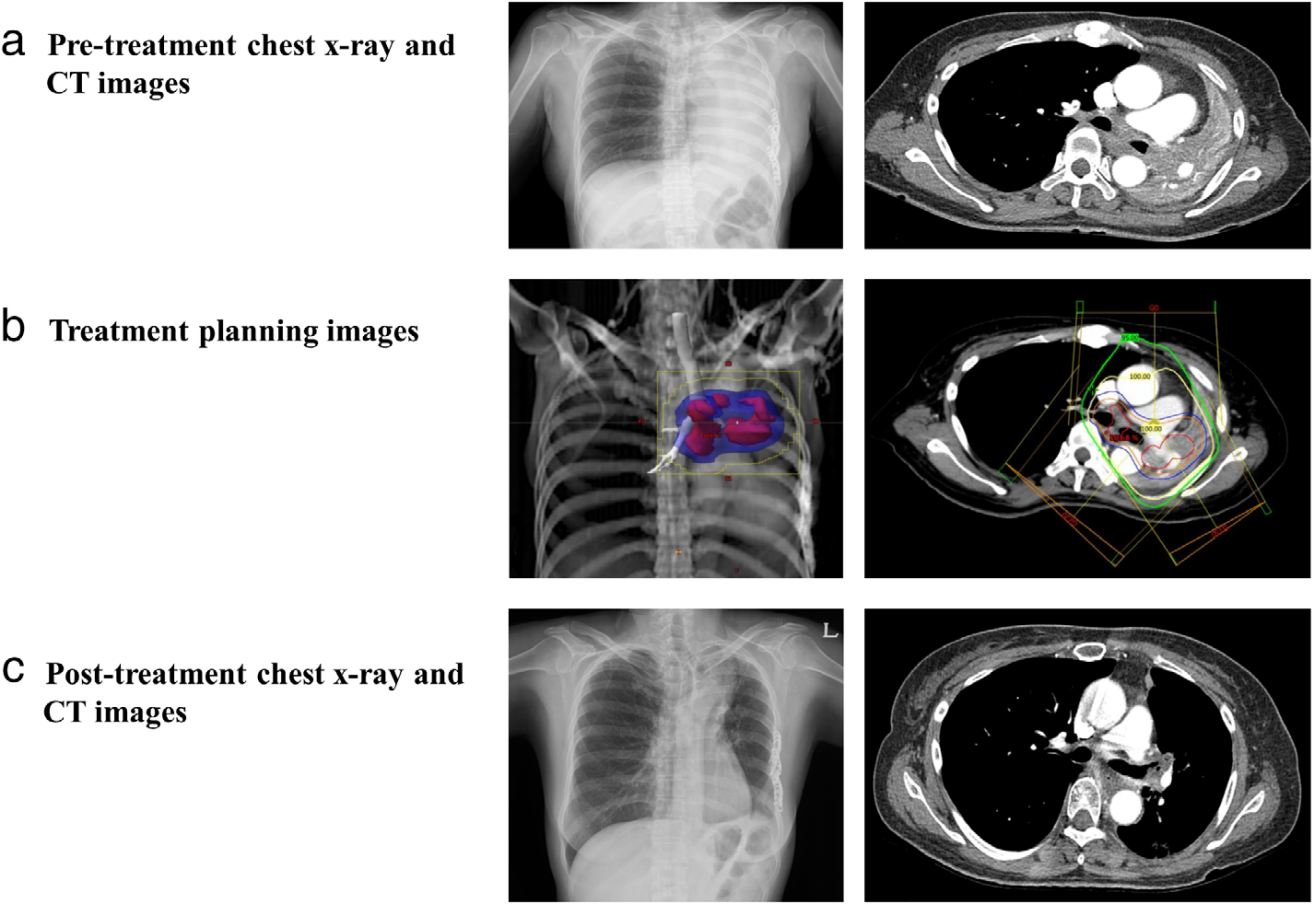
Images for a patient who underwent palliative radiotherapy for malignant airway obstruction. a Pretreatment chest X‐ray and CT images; b Treatment planning images; and c Post‐treatment chest X‐ray and CT images.
